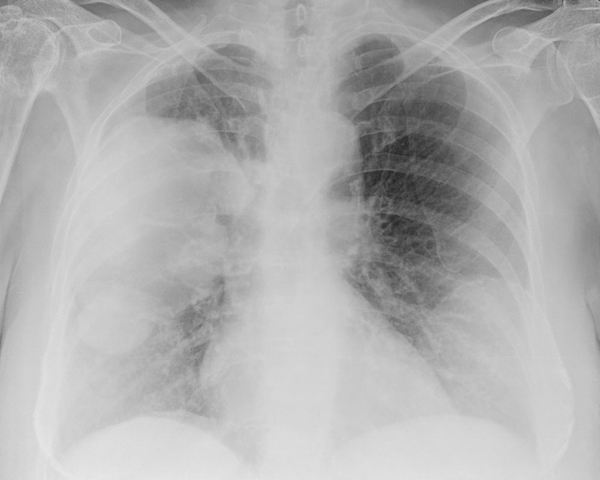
Chest X-ray showing a large opacity in the right hemithorax.
